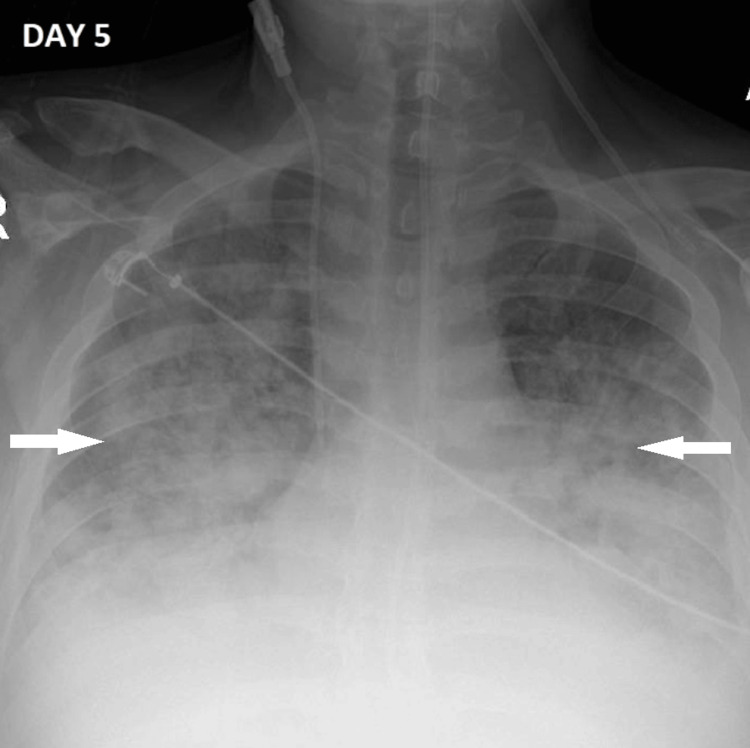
Anteroposterior chest radiograph obtained on day 5, prior to reintubation Demonstrating marked worsening of bilateral pulmonary infiltrates and airspace opacification (white arrows) consistent with cardiogenic pulmonary edema. Nasogastric tube, central venous catheter, and peripheral lines are in situ.
